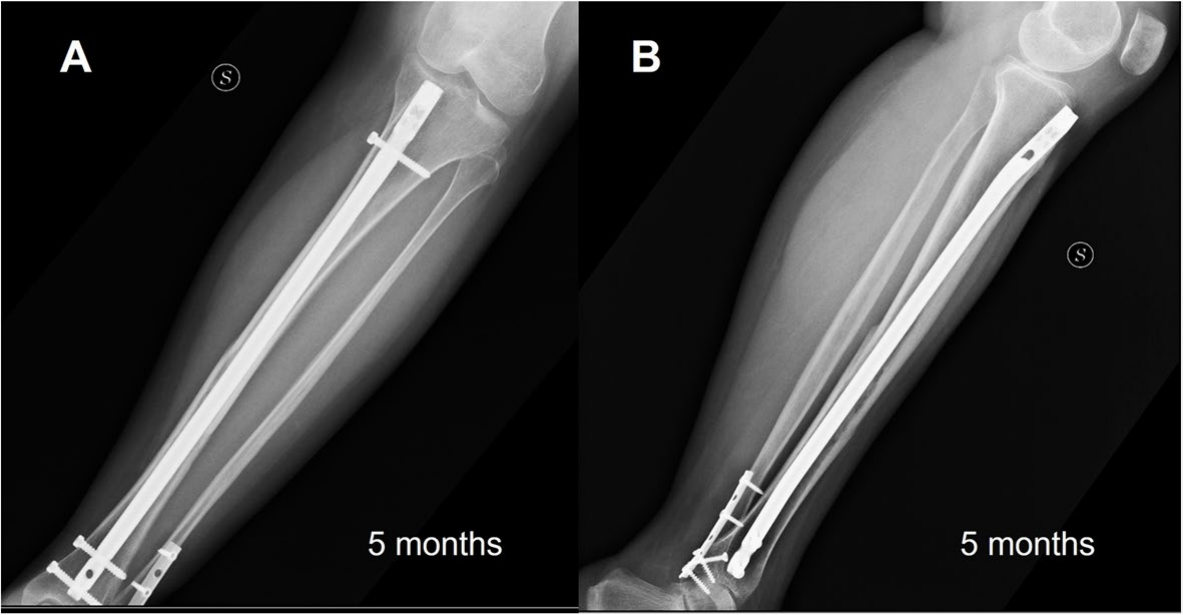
Plain radiograph of the left leg at 5 months follow-up
